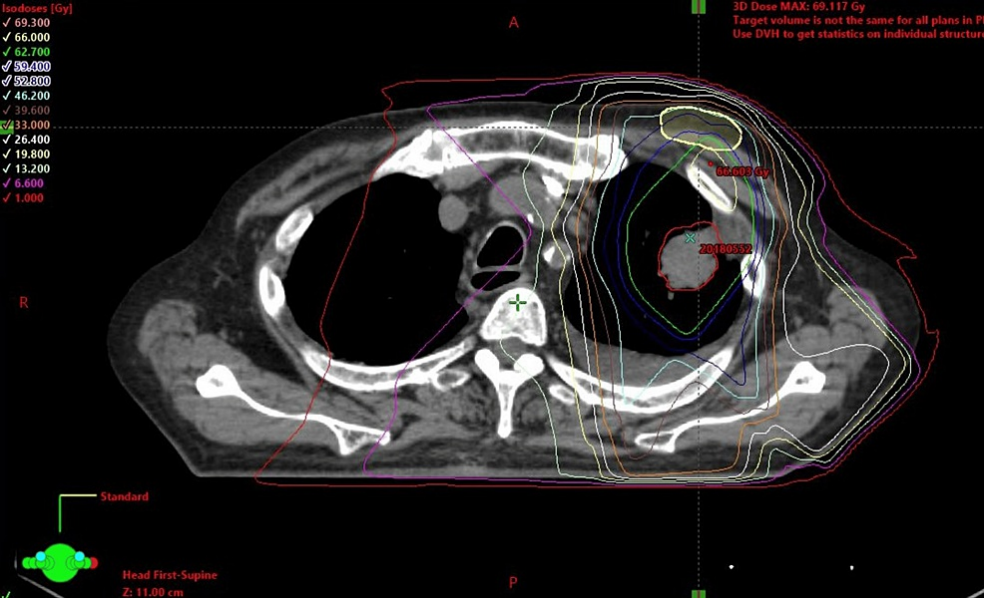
CT before radiation therapy. Estimated irradiation to the pacemaker exceeds 66 Gy.
